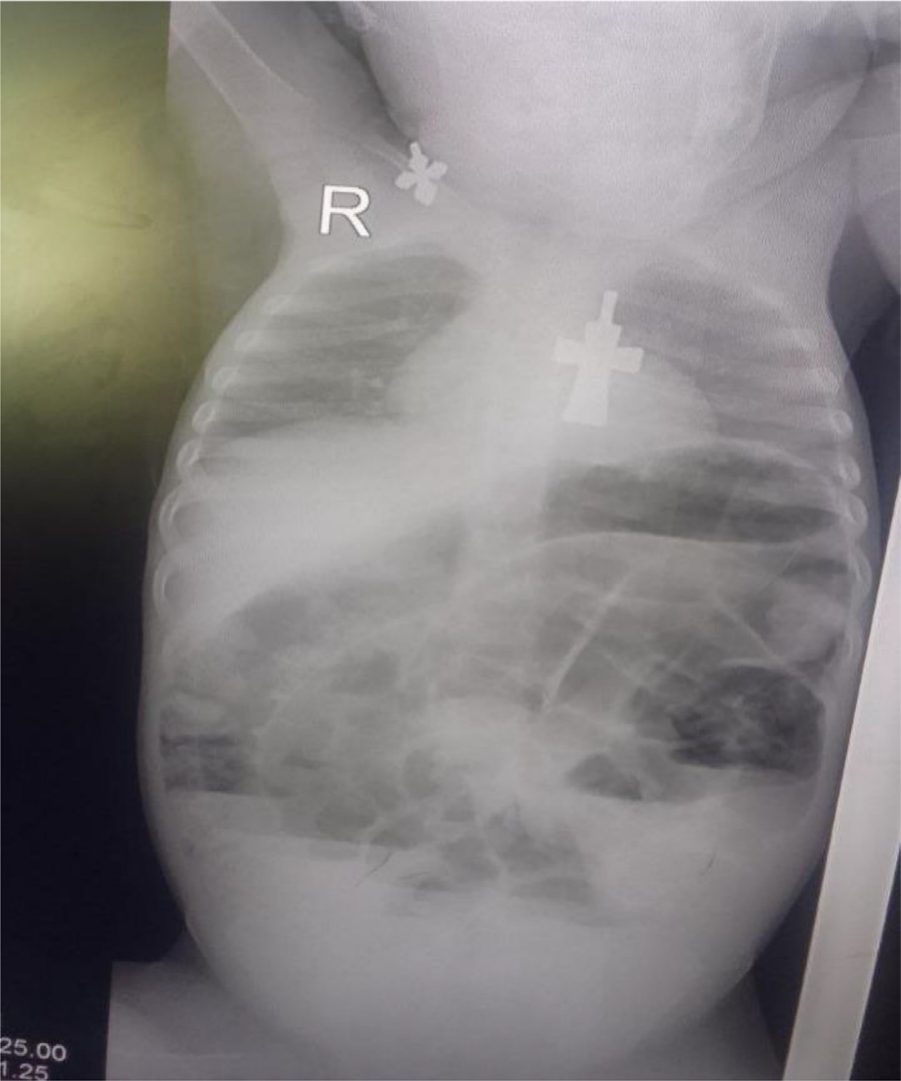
Plain abdominal X-ray shows multiple air-fluid levels with dilated bowel loops and absent rectal air shadow.
